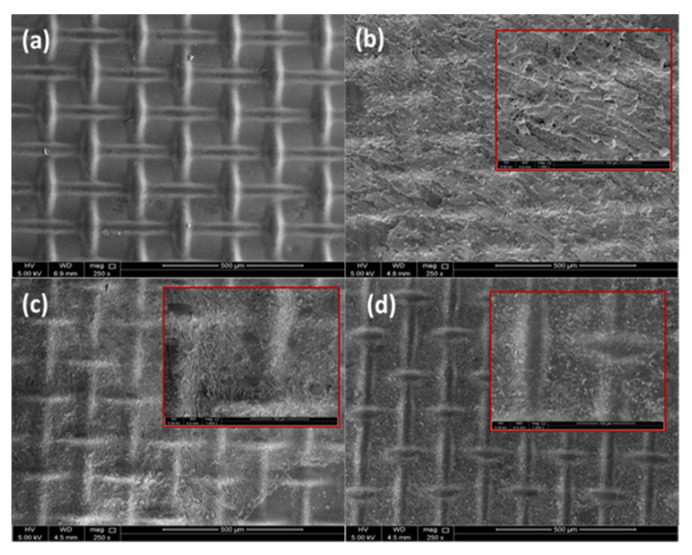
Cross-sectional SEM image of the active layer surface of the CTA FO membrane after three consecutive FO runs: l (a) origin; (b) without physical cleaning; (c) in situ flushing; and (d) osmotic backwash. Conditions: FS = SWW, DS = MCFDS (300 g/L) and FS and DS flowrate = 1.6 LPM at room temperature for 24 h operating time.
